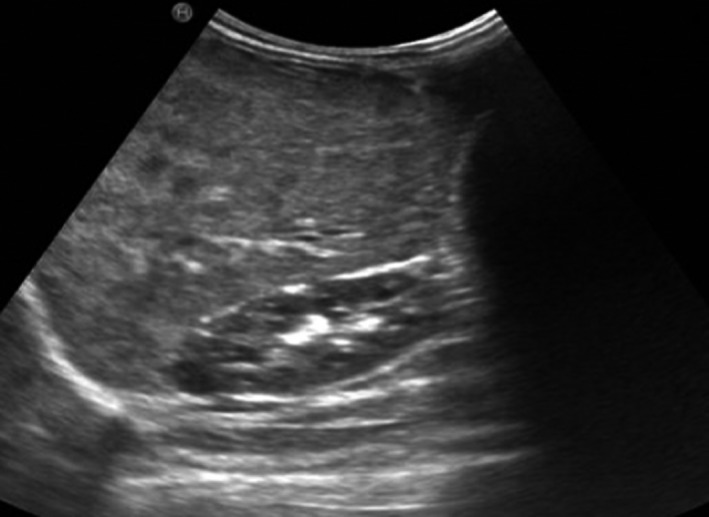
Abdominal ultrasonography showing reduction in liver size and hemangiomas. More liver parenchyma is seen.
